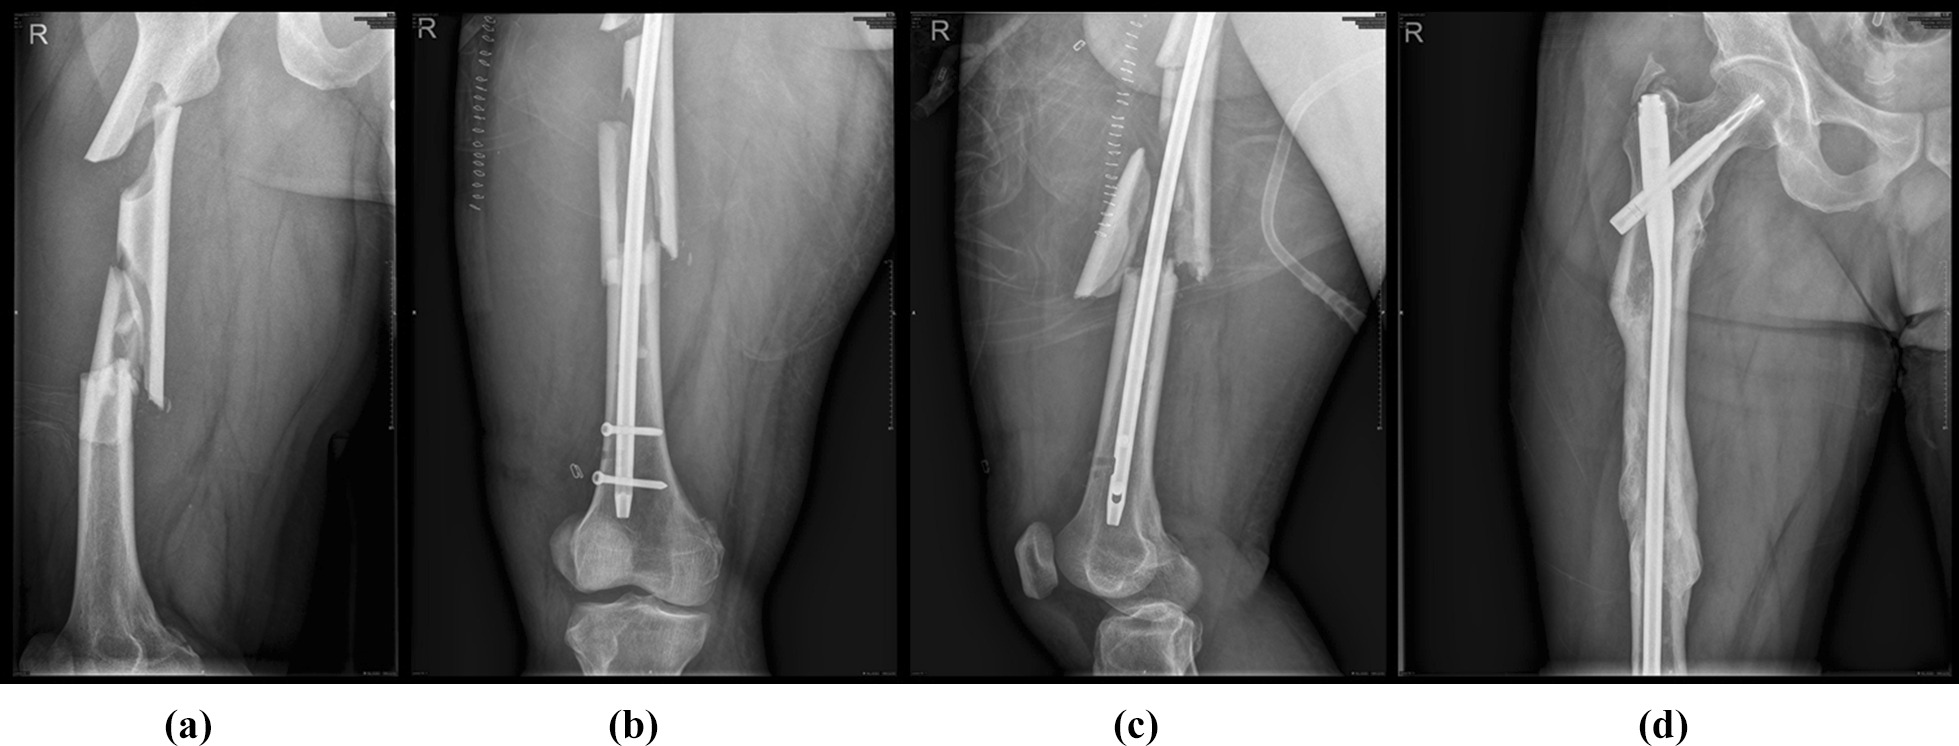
a A comminuted femoral shaft fracture with displaced major fragments. b After 13 days, intramedullary nailing was performed to stabilize the comminuted femoral shaft fracture with residual displaced major fragments on the anterior–posterior image. c Displacement of the major fragments was more pronounced (> 1 cm) on the lateral image. d After a period of 24-month follow-up, the X-ray image showed bone union of the femoral shaft fracture with displaced major fragments
